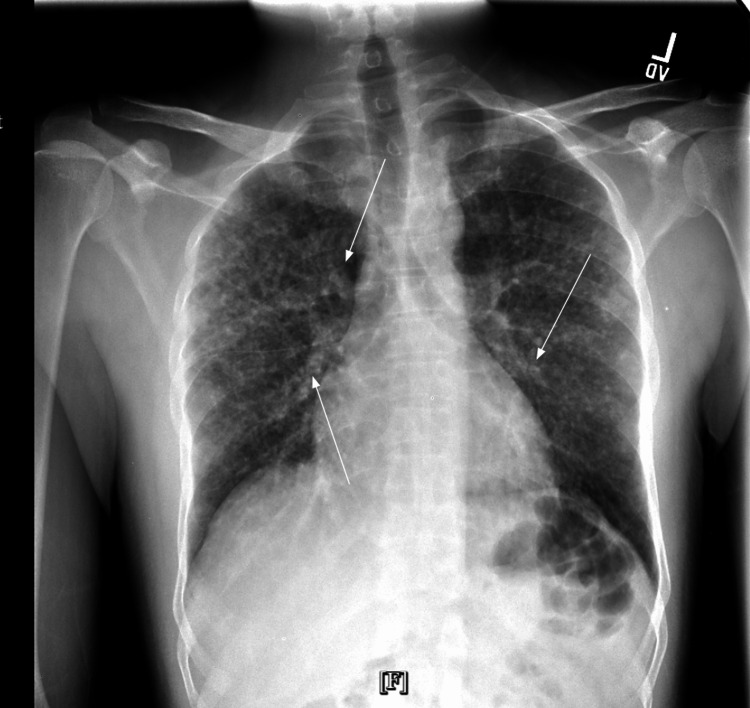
Diffuse, bilateral granular opacities (white arrows) in the chest X-ray
